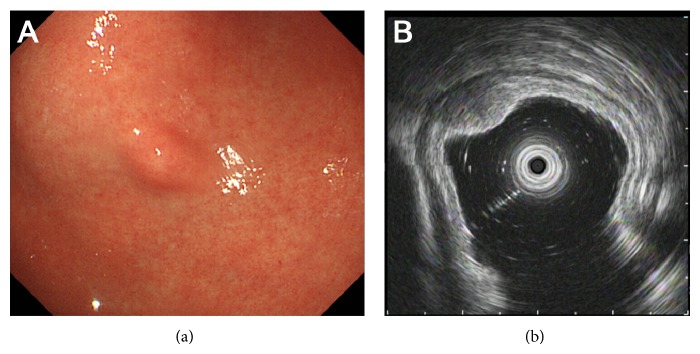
Endoscopic images. Esophagogastroduodenoscopy revealed a submucosal tumor with a diameter of approximately 10 mm in the greater curvature of the gastric antrum (a). Endoscopic ultrasonography showed a solid tumor with hypoechoic internal echogenicity measuring 11.8 mm, which was located in the deep mucosal and submucosal layers (b). Anechoic duct-like structure was not detected in the tumor.
